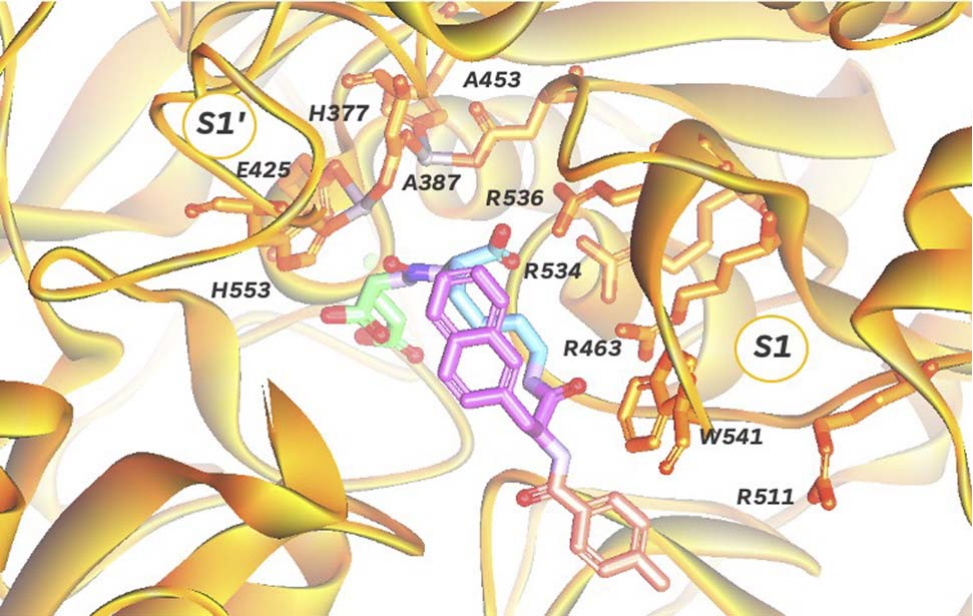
Depiction of PSMA dinuclear zinc active site cavity (orange, PDB: 5O5T) with a bound PSMA617 ligand, consisting of a glutamate (green)-urea (purple)-lysine (blue)-napthylalanine (pink)-tranexamic acid (pale red) motif.
